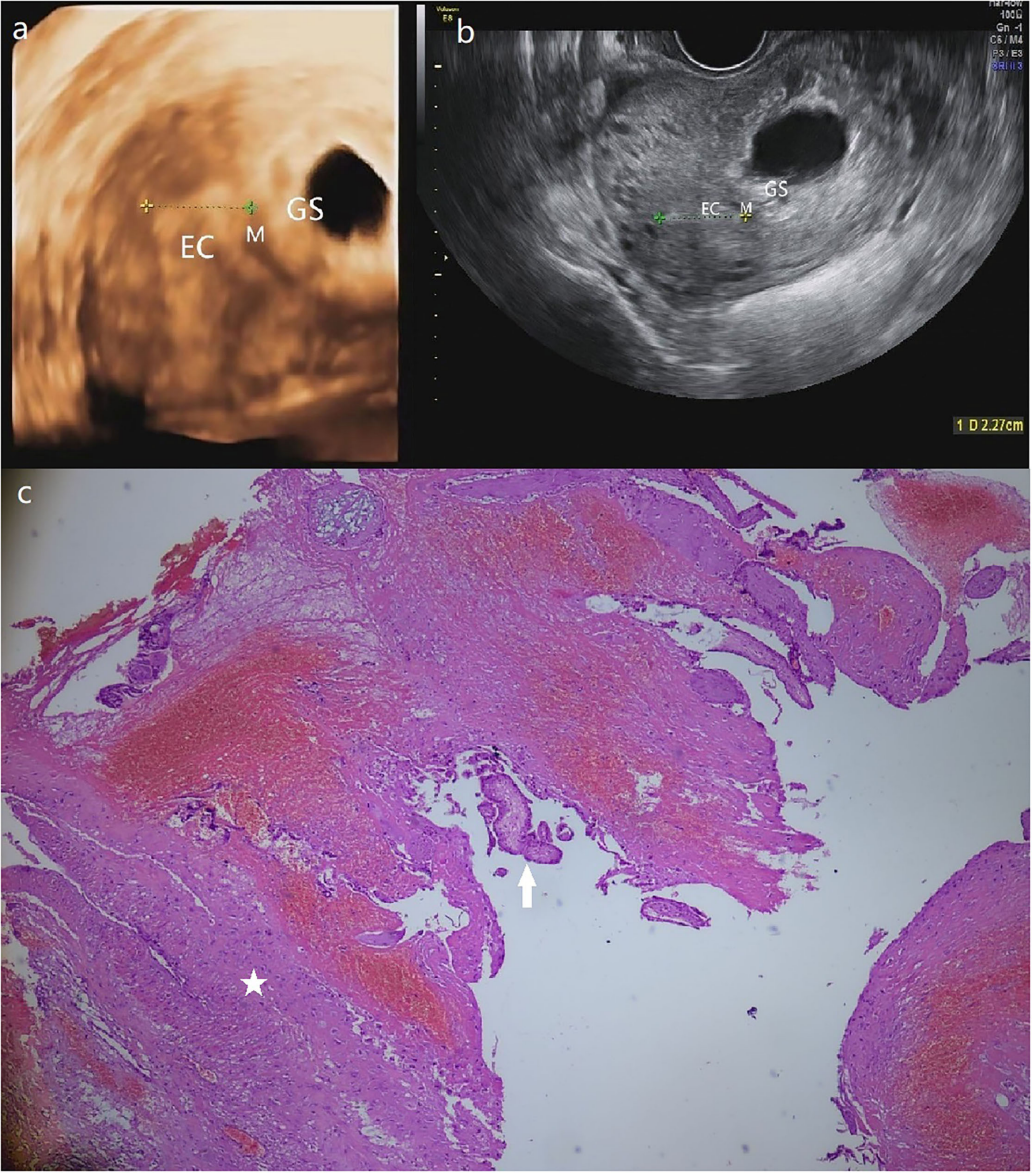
(a) The GS in a size of 4.9*3.4 cm is detected in a heterogeneous echo mass of 6.1*4.5 cm at the left posterior uterine wall near the left corner of the 3-D ultrasound. (b) Transversal section image in 2-D ultrasound demonstrates the intramural pregnancy and shows that the GS has no communication with EC. (c) The pathological result shows the myometrium (star) and the chorionic villus (arrow). (Patient 3, GS, gestational sac; M, myometrium; EC, endometrial cavity).
